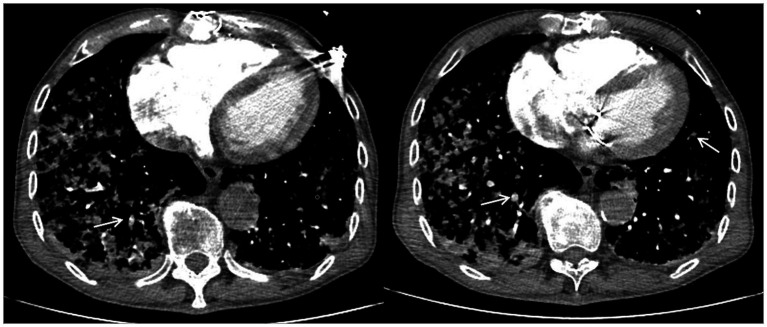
Computed tomography pulmonary angiography showing multiple pulmonary artery branch embolisms in both lungs. White arrows indicate pulmonary artery branch thrombosis.
